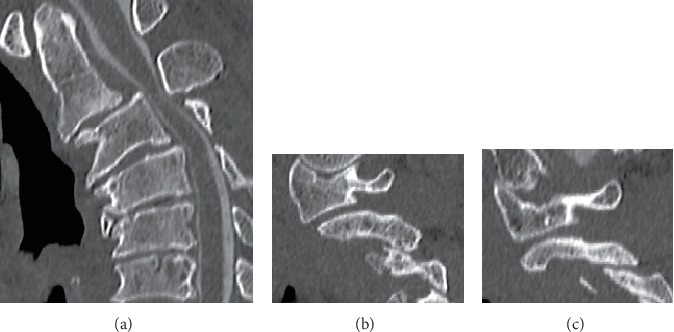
Preoperative CT myelography images. (a) Sagittal view. (b) Right view and (c) Left view of the C2-3 facet joint. CT images show that the bilateral pars interarticularis were not fractured.
